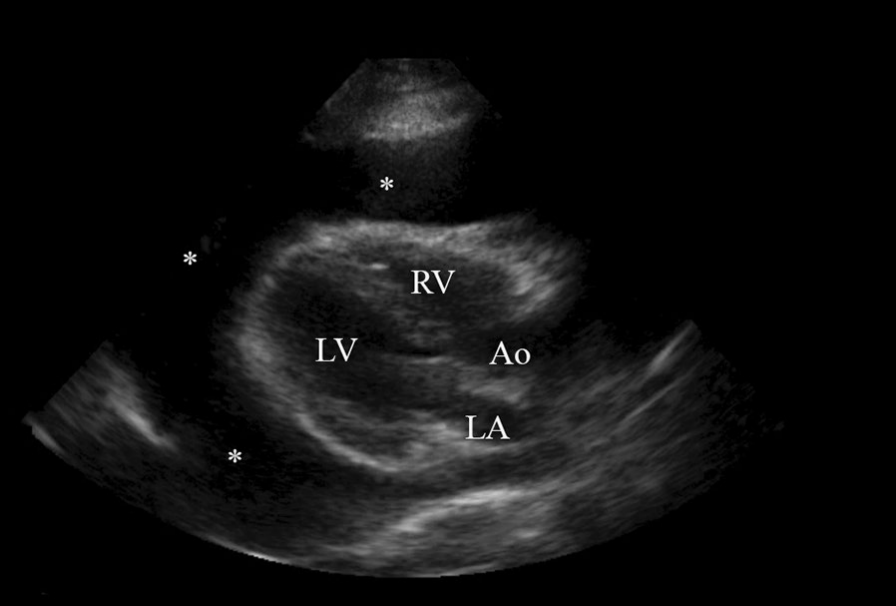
POCUS, Case 1. Parasternal long-axis view of pericardial tamponade with a large circumferential pericardial effusion (*). The classic “swinging heart” was visualized in real-time ultrasonography. For orientation, the “emergency medicine” orientation was used for scanning. Structures visualized are: the left ventricle (LV), right ventricle (RV), left atrium (LA) and aortic outflow tract (Ao)
